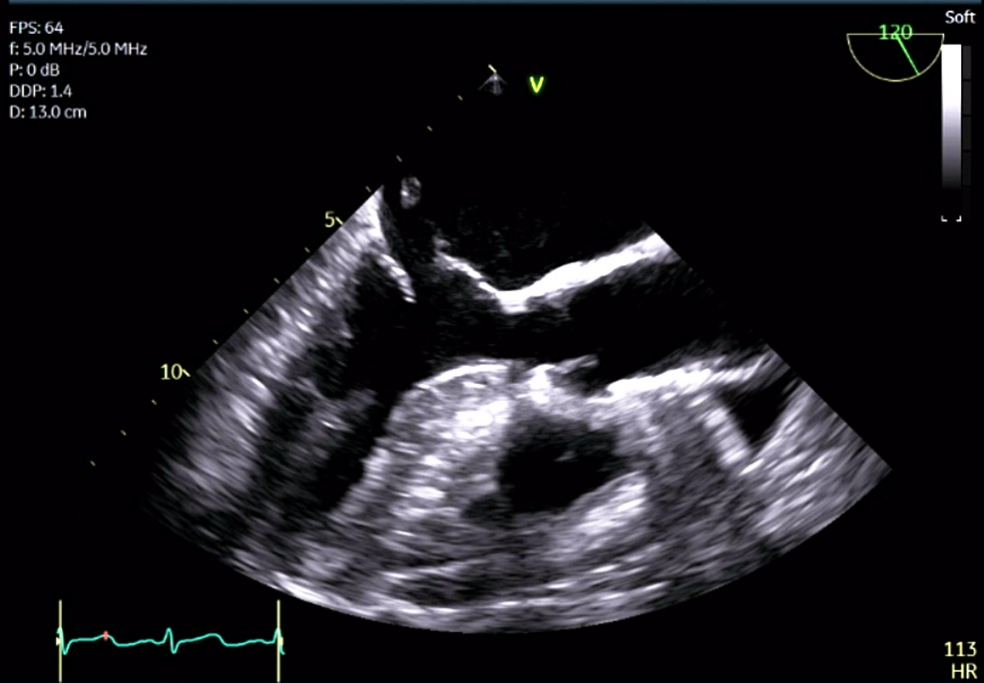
Transesophageal echocardiogram showing flail anterior mitral valve leaflet during systole with the ruptured anterior papillary muscle attached to the chordae tendineae.
